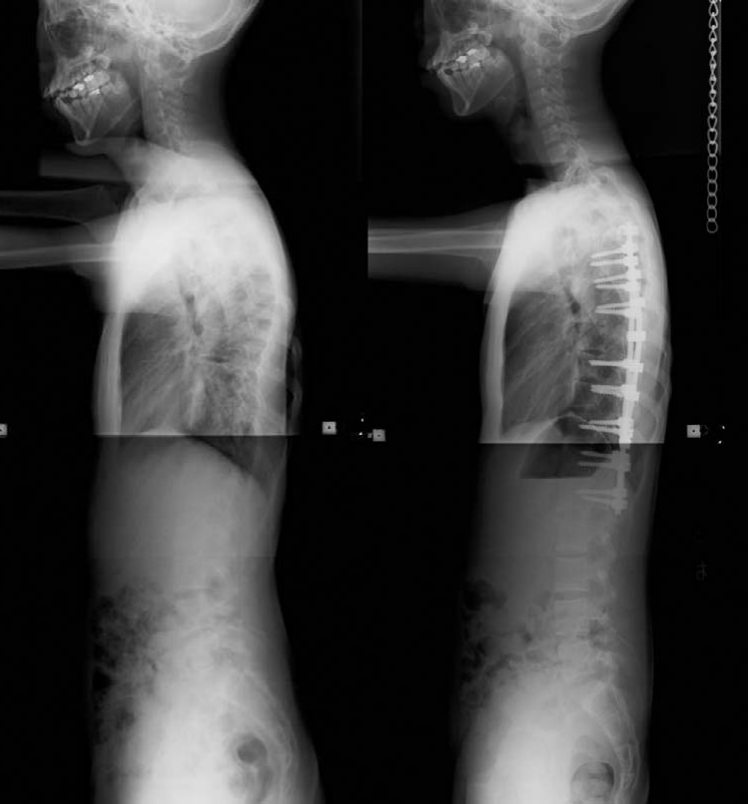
Segmental pedicle screw constructs. Lateral radiographs before and after surgery.
